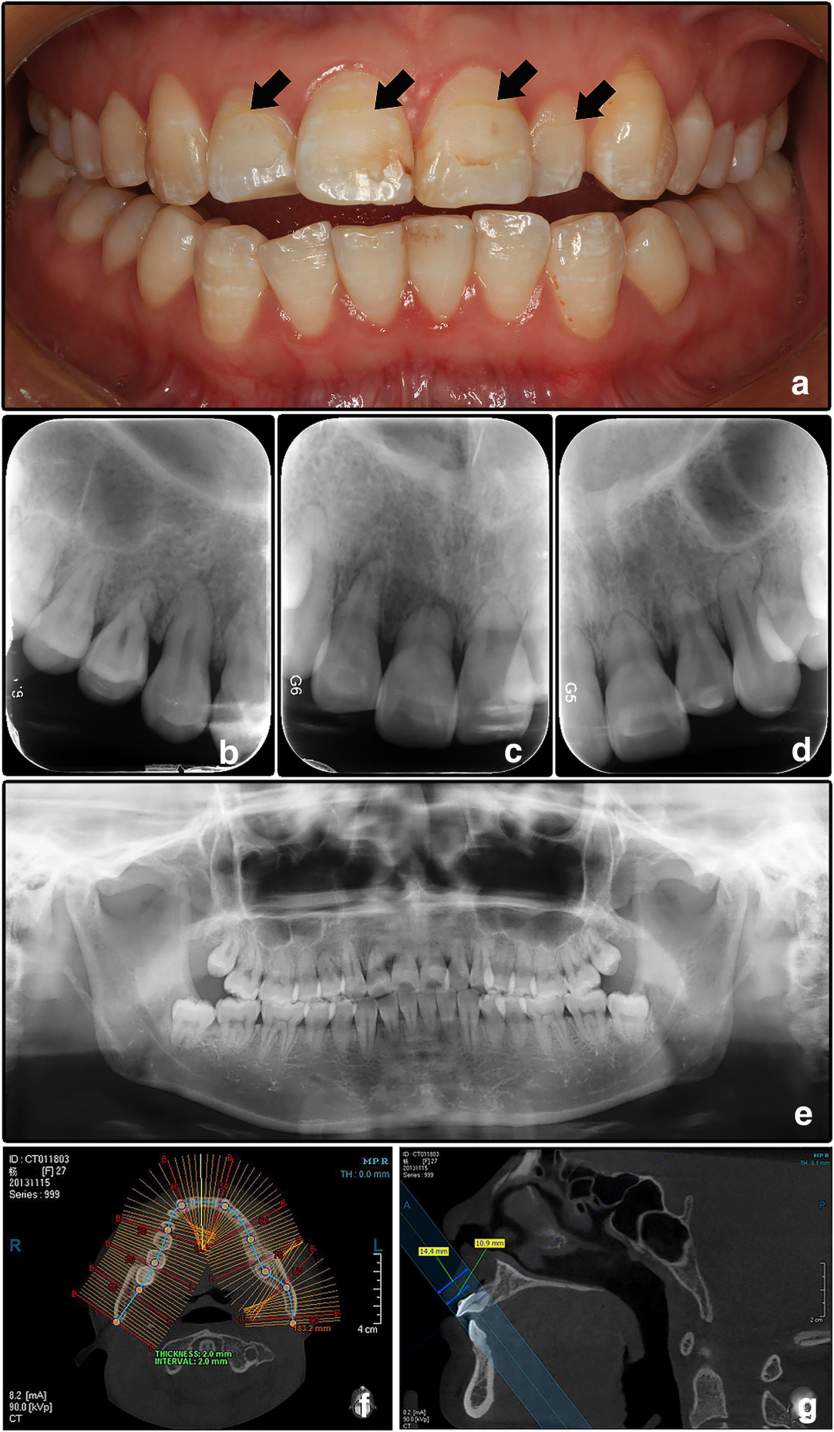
Clinical and imaging manifestation of Case 1. a Frontal intraoral view shows the teeth crowns appear normal except for several spots due to mild enamel hypoplasia. b-d Periapical radiographs show the short root anomaly of the maxillary incisors. e Panoramic film. f-g Teeth lengths were measured by CBCT
